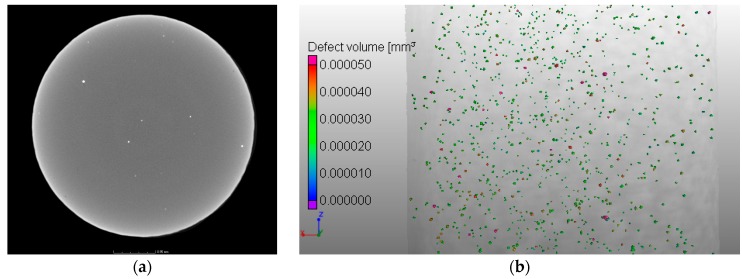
X-ray CT images of a contaminated (Batch 1) tensile sample built in Z direction showing (a) high density inclusions (white particles); and (b) distribution of these defects in the part, coloured according to their associated volume.
